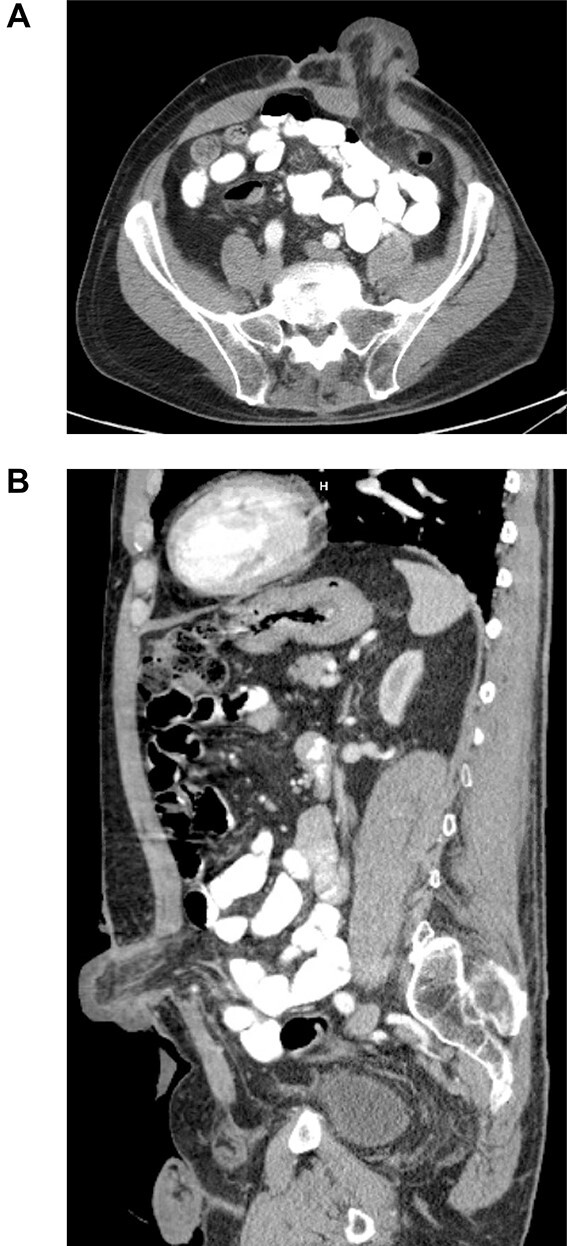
Axial (A) and sagittal (B) CT of the abdomen and pelvis, with IV contrast demonstrating edematous colostomy above the level of the fascia.
